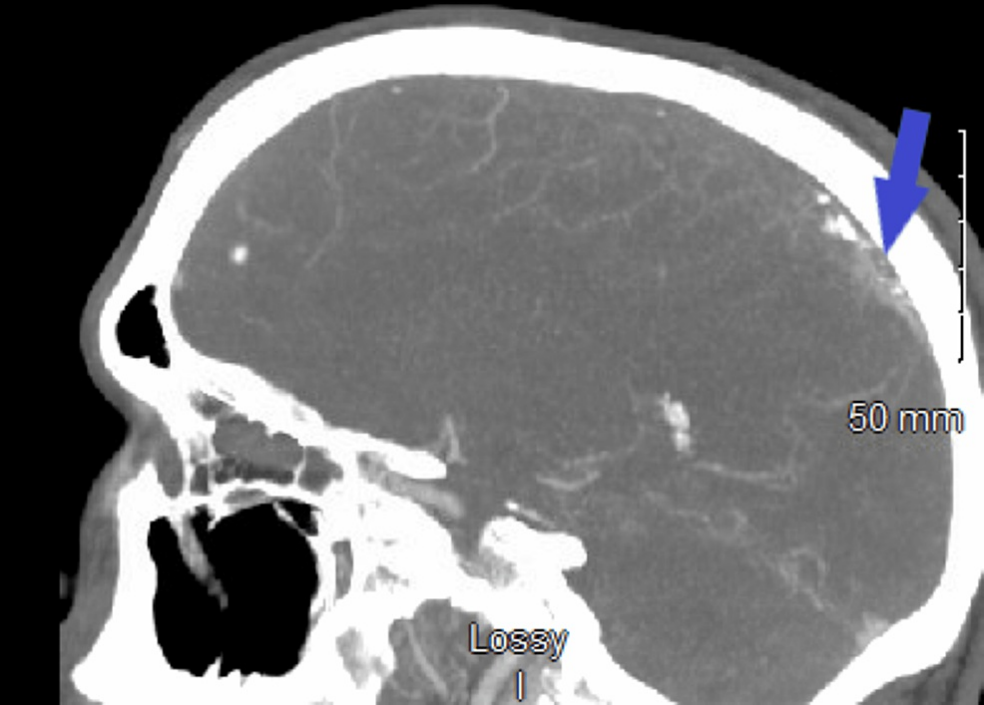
Computed tomography angiography of the head (sagittal view). The blue arrow shows superior sagittal sinus thrombosis.
